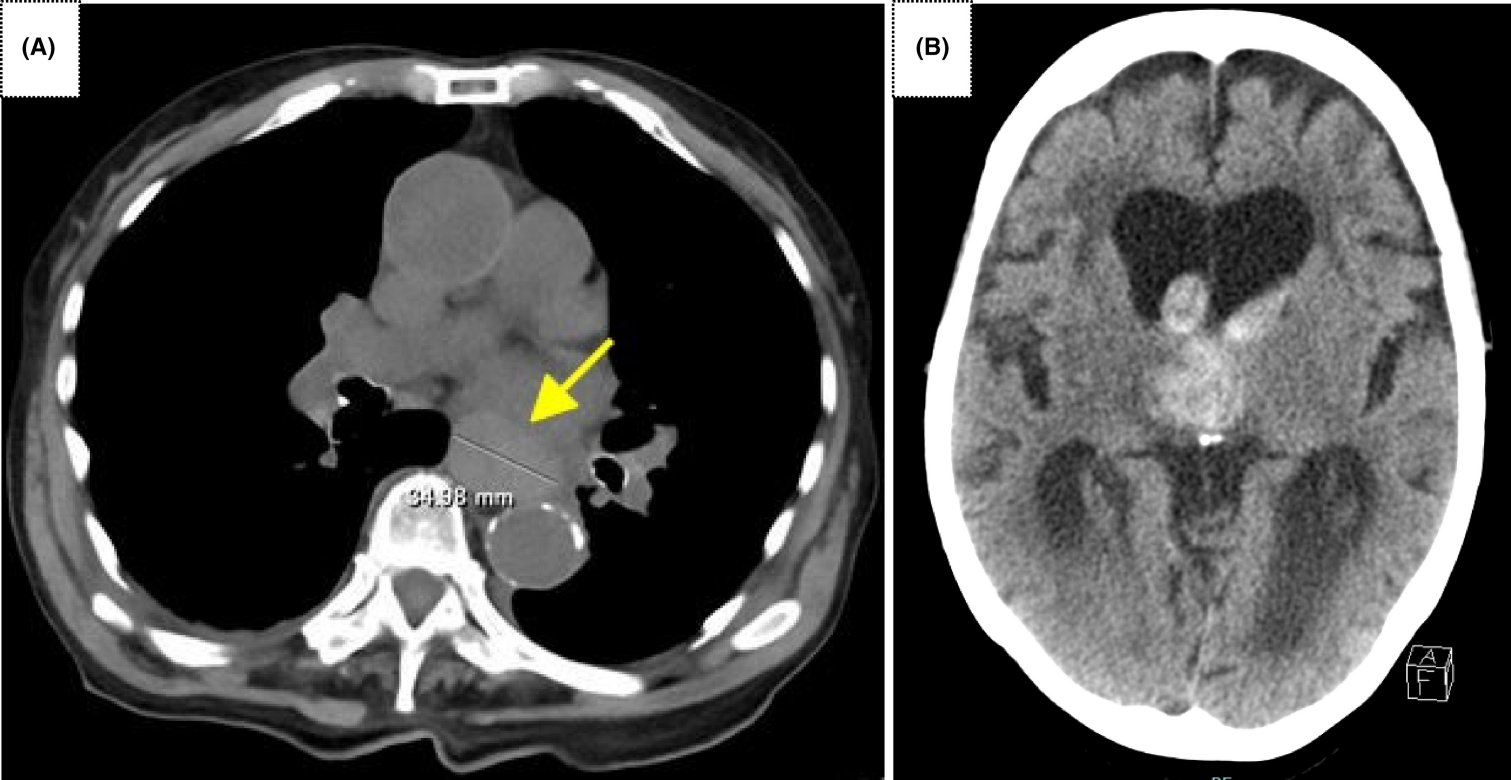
(A) Simple chest computed tomography (CT) scan showing a 35‐mm mass in the esophagus. Due to the presence of a hiatal hernia of the esophagus, the tumor was found to be more cephalad than its original location. (B) Simple head CT scan showing right thalamic hemorrhage and perforation of the lateral ventricle.
